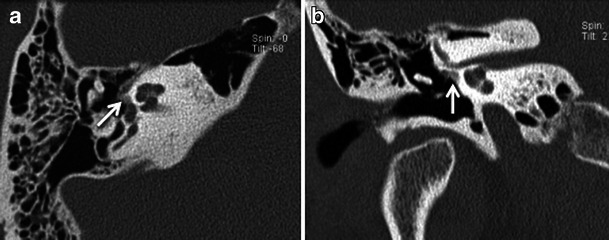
Axial (a) and coronal (b) HRCT images of the right temporal bone in an adult patient with right-sided CHL. A hypodense demineralised plaque (*arrow*) is noted in the region of the fissula ante fenestram in keeping with fenestral otosclerosis
